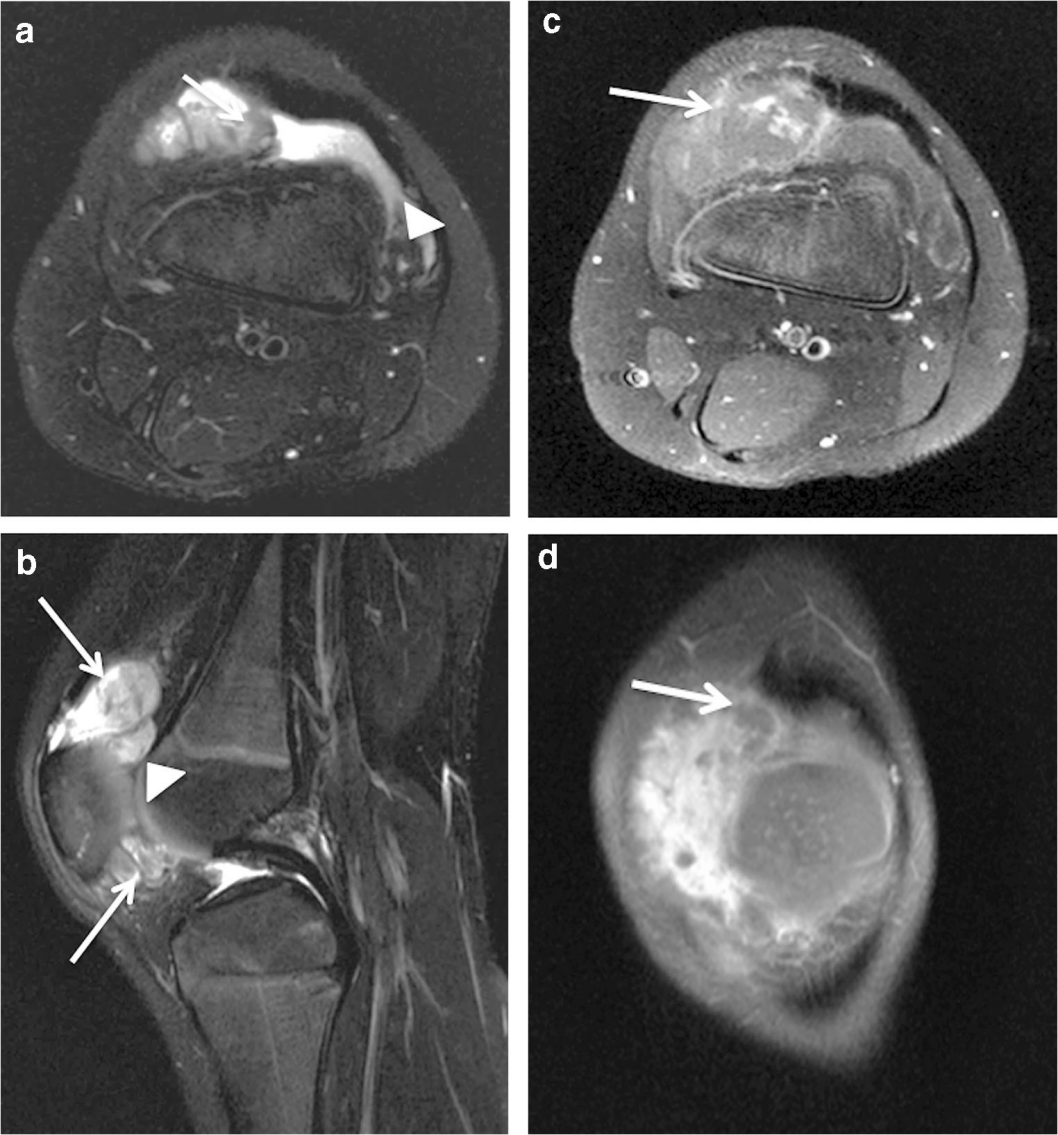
A 7-year-old boy with an intraarticular and intrasynovial venous malformation of the knee. a, b Axial (a) and sagittal (b) T2-weighted fat-saturated MR images. The cluster-like venous malformation protrudes into the suprapatellar recess (*arrow* in a). A mild synovial effusion with post-hemorrhage debris is present (*arrowhead* in a). The venous malformation invades both the suprapatellar and infrapatellar fat pads (*arrows* in b) as well as the intrasynovial cavity (*arrowhead* in b). c, d T1-weighted fat-saturated post-contrast axial (c) and coronal (d) MR images demonstrate the progressive enhancement typical for venous malformations. The first post-contrast image (c) shows only mild and partial enhancement of the venous malformation (*arrow*). A consecutively obtained coronal image (d) demonstrates more extensive enhancement. The filling defects (*arrow* in d) are due to intraluminal thrombosis often present in venous malformations
